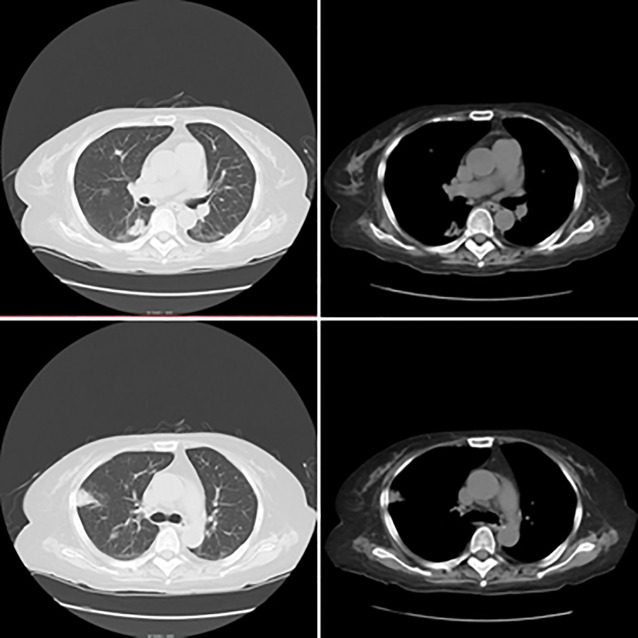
Chest CT scan before admission. The scan demonstrates bilateral interstitial pneumonia along with multiple pulmonary nodules and mass-like opacities. Comparison with the prior examination shows interval improvement and partial resolution of these opacities.
